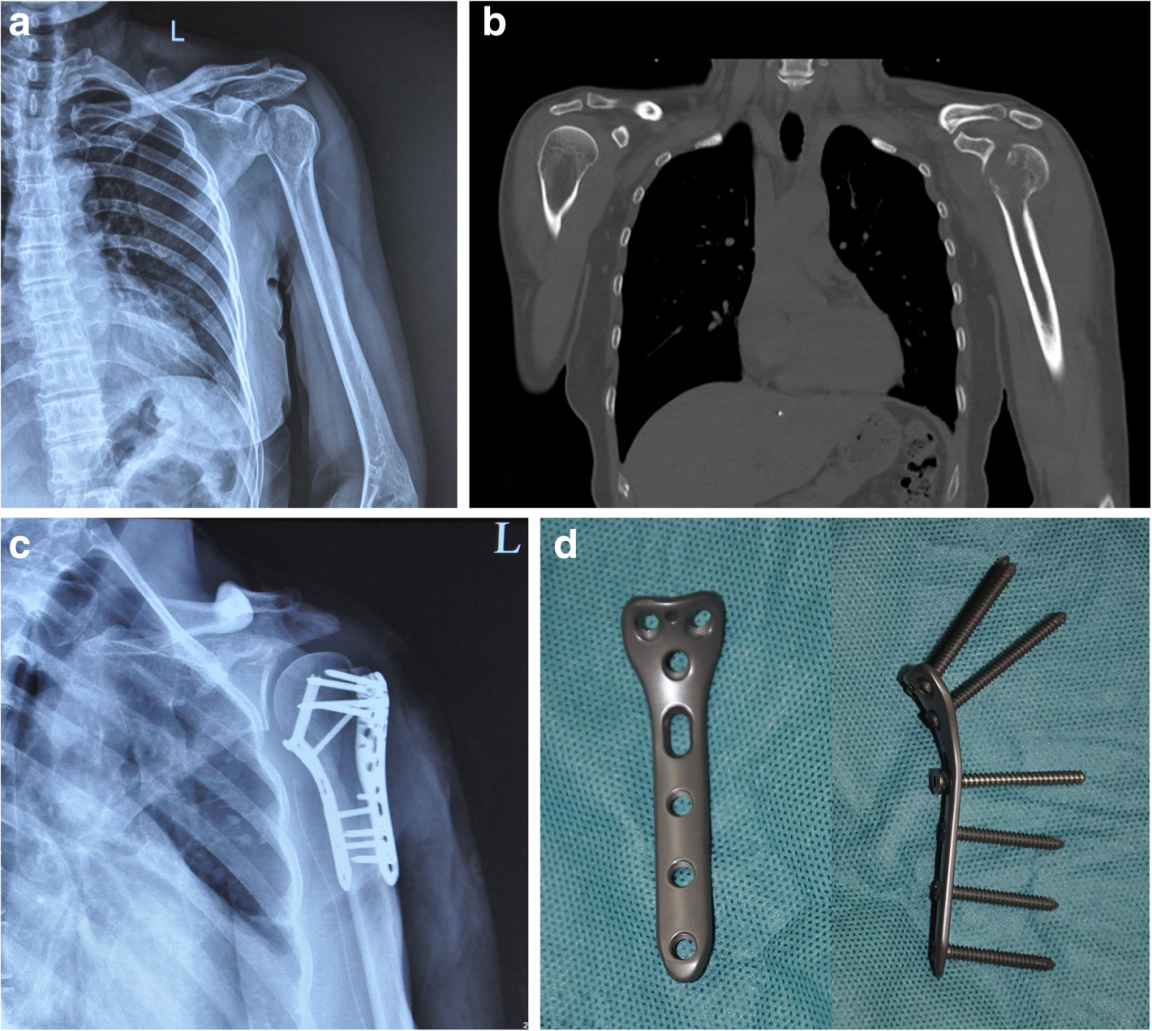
A 45-year-old female patient sustained a proximal humeral fracture with posterior dislocation of the shoulder joint. Preoperative anteroposterior radiographs (a) and computed tomography (b) scanning were performed. The medial column of the humerus was unstable (b). The postoperative anteroposterior radiograph (c) showed the application of the LPMP method (d)
